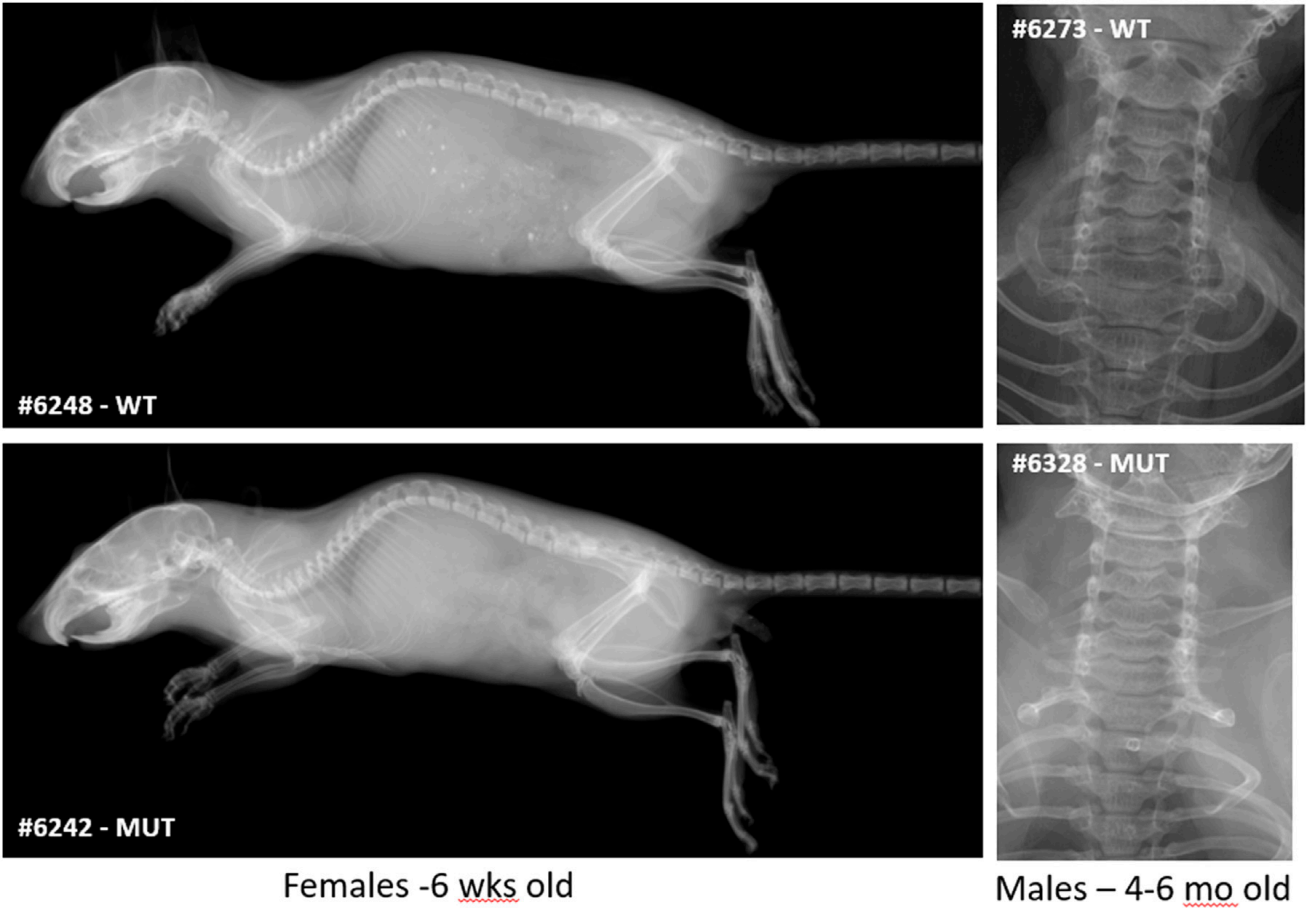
Skeletal survey. X-ray images at either 6 weeks (full skeleton) or 4–6 months of age (upper thoracic and neck area) did not show significant macroscopic differences or cervical vertebral malformations typical of SMC2.
